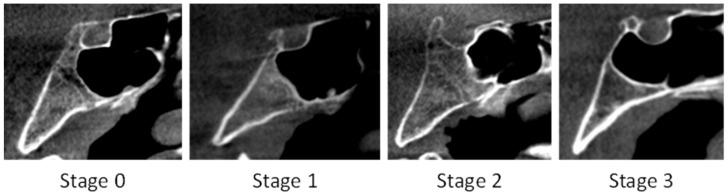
CBCT scans showing the SOS fusion stages (Stages 0–3).
